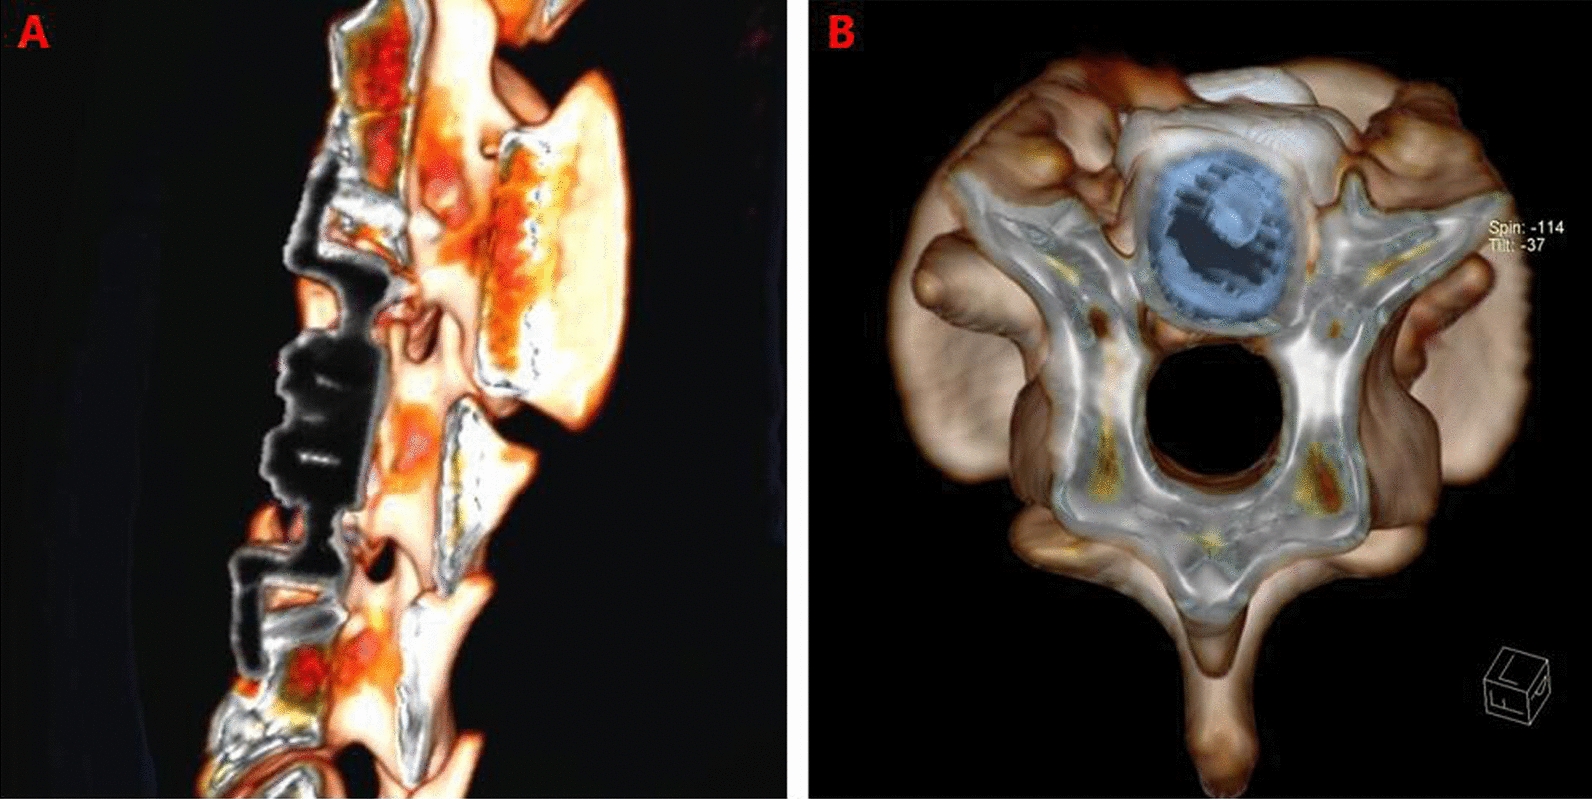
3D CT reconstruction 3 months after surgery. A, Sagittal view. B, Axial view
